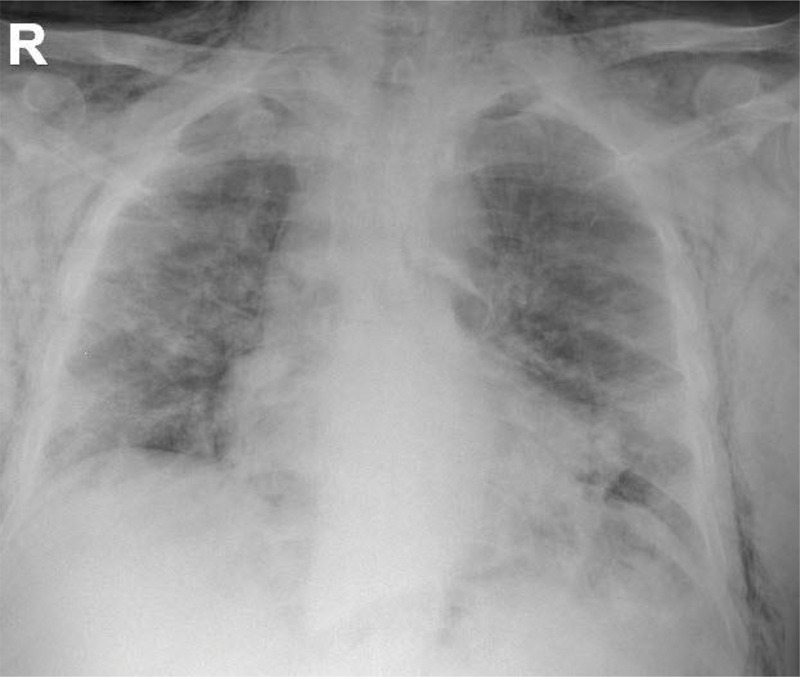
Diffuse subcutaneous emphysema and bilateral parenchymal diffuse ground glass appearance on posteroanterior chest radiography.
